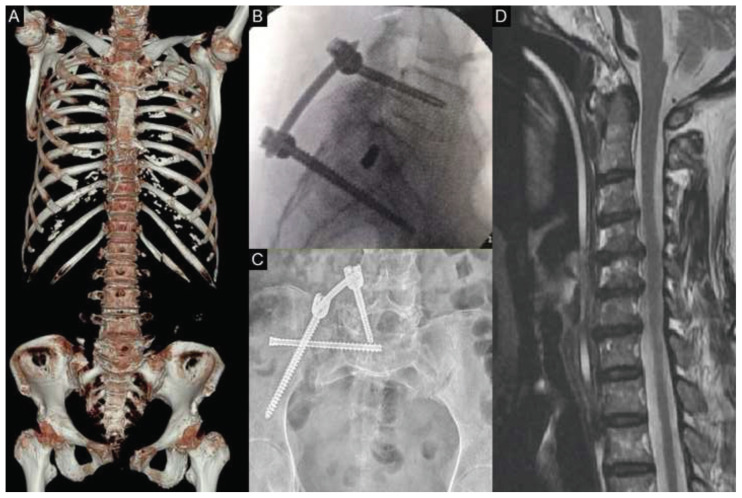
(A) Chest–abdomen–pelvis CT showing fractures of the left 4th, 5th, 11th, and 12th ribs, and complex pelvic fractures involving the right sacrum, right iliac wing, and bilateral pubic rami. (B) Intraoperative sagittal fluoroscopic view demonstrating placement of right L5-S2-alar-iliac screw fixation (6.5 × 50 mm and 7.5 × 90 mm). (C) Postoperative pelvic radiograph showing stabilization of the sacral fracture with a 7.0 × 90 mm Aspire headless screw. (D) Sagittal magnetic resonance imaging (MRI) of the cervical spine demonstrating pronounced multilevel bilateral foraminal stenosis from C4 to C7.
